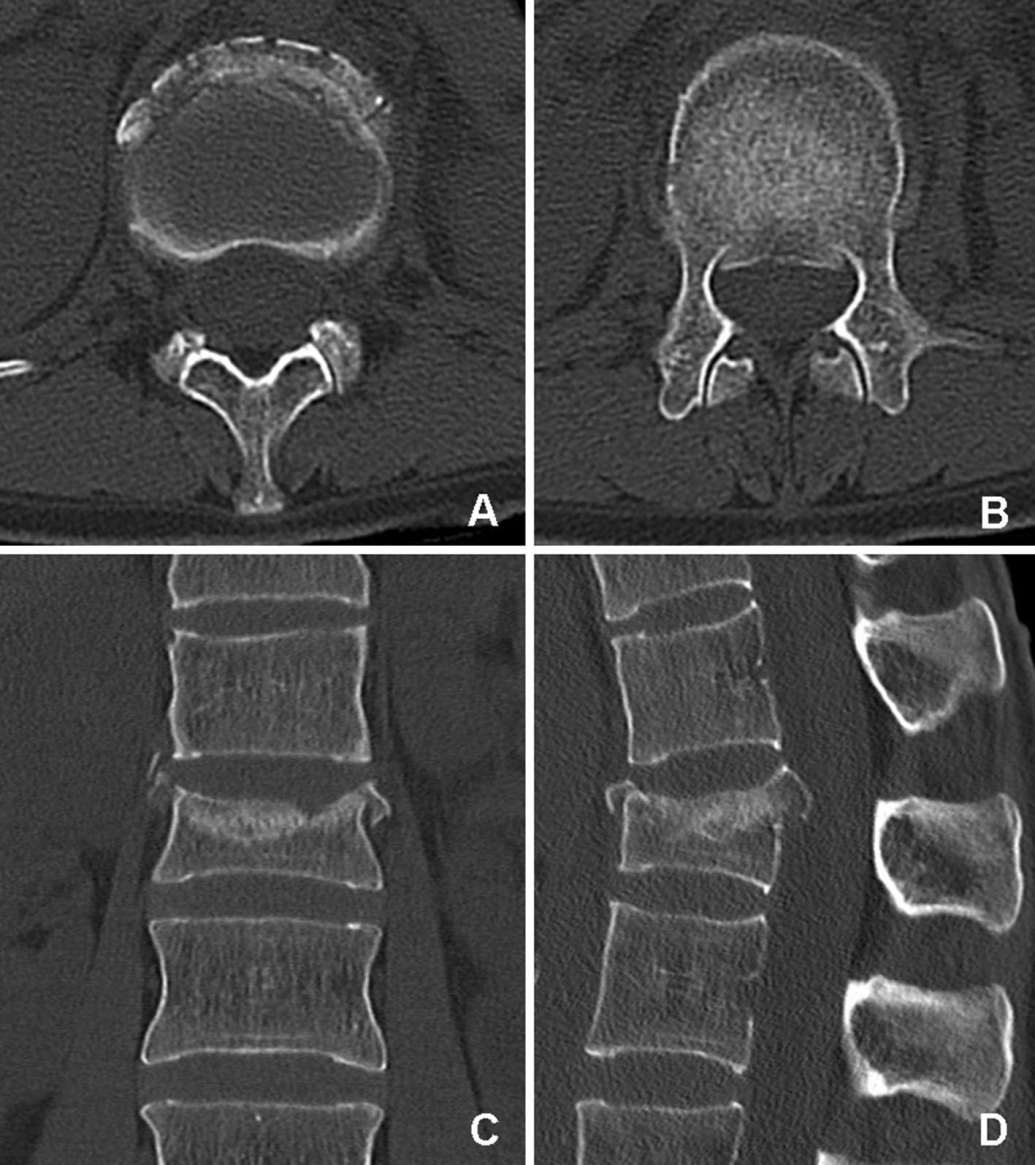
Preoperative CT of a 42-year-old woman with a superior incomplete burst fracture (Magerl et al.^19^ A3.1.1, AOSpine A3^1^, load sharing classification score 7^3^) of L1 sustained in a car accident. CT scans showing representative axial (A and B), coronal (C) and sagittal (D) slices of the thoracolumbar junction. *The upper half of the vertebral* body was partially comminuted with centrifugal extrusion of fragments. Fragments of the posterior wall are retropulsed into the spinal canal.
